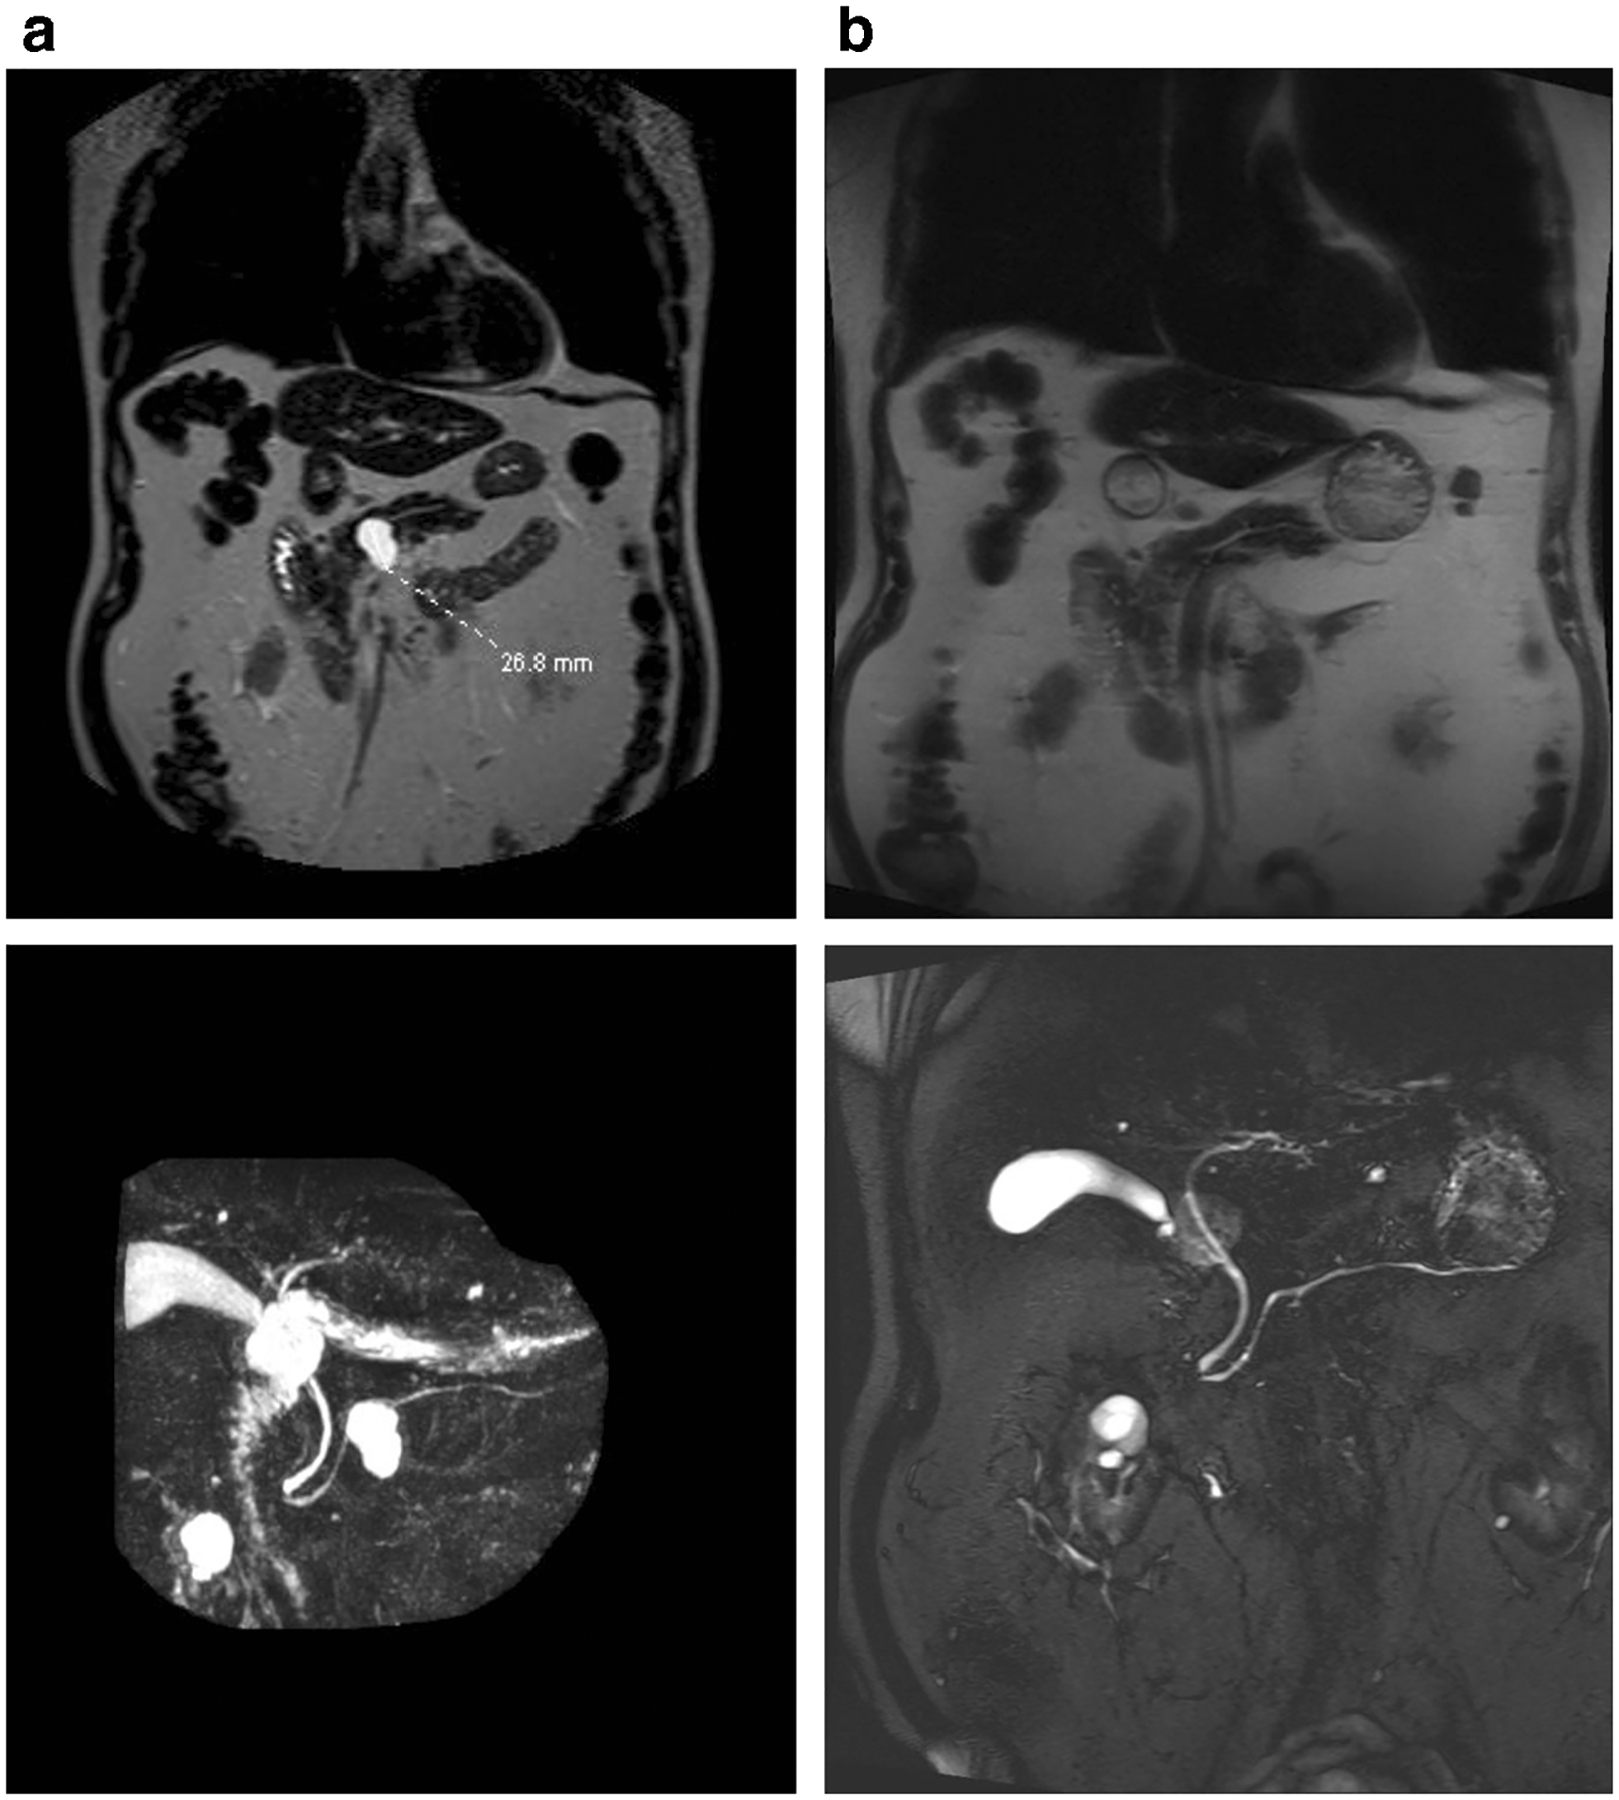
a MRI-MRCP imaging of an 83-year-old male with a 2.7-cm intraductal mucinous pancreatic neoplasm (white arrow) prior to EUS-guided chemoablation. b Follow-up MRI-MCRP imaging reveals no evidence of residual cyst at 6 month follow-up evaluation
